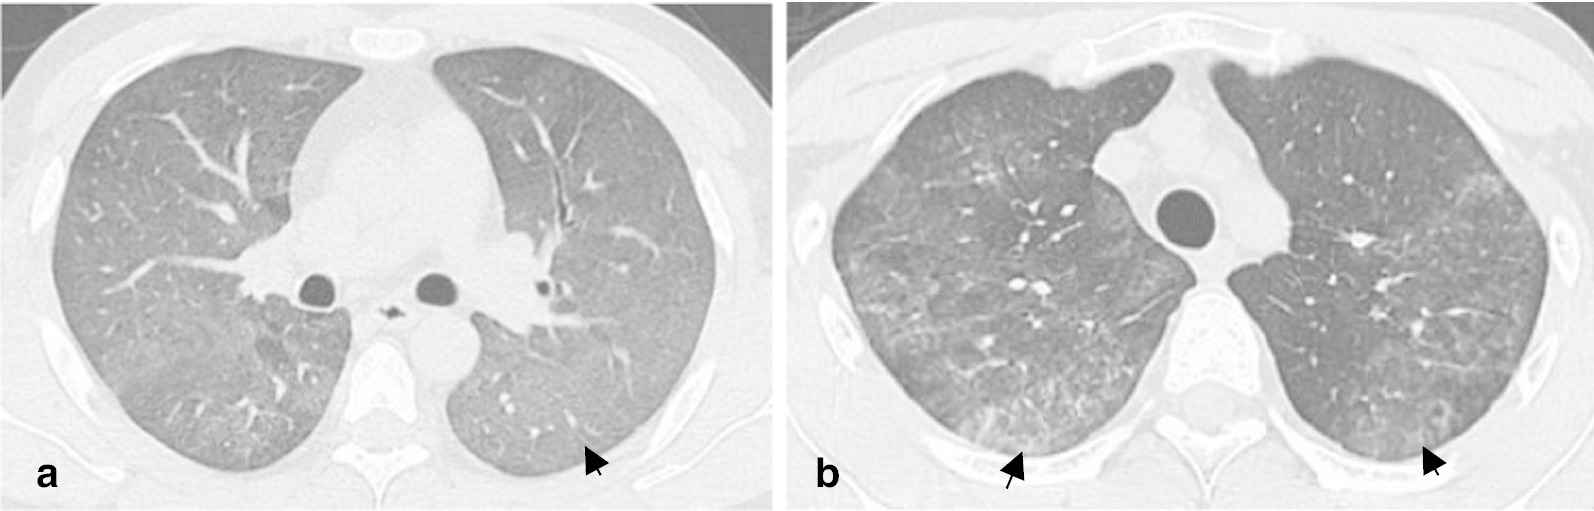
Pneumocystis jirovecii* pneumonia. a Pneumonia caused by *P. jirovecii* in a 28-year-old male patient with AIDS. HRCT image showed diffuse ground-glass opacity of bilateral pulmonary parenchyma (arrow). b Pneumonia caused by *P. jirovecii* in a 31-year-old male patient with AIDS. HRCT image showed multiple ground-glass opacity of bilateral pulmonary parenchyma with reticulate changes (arrows)
